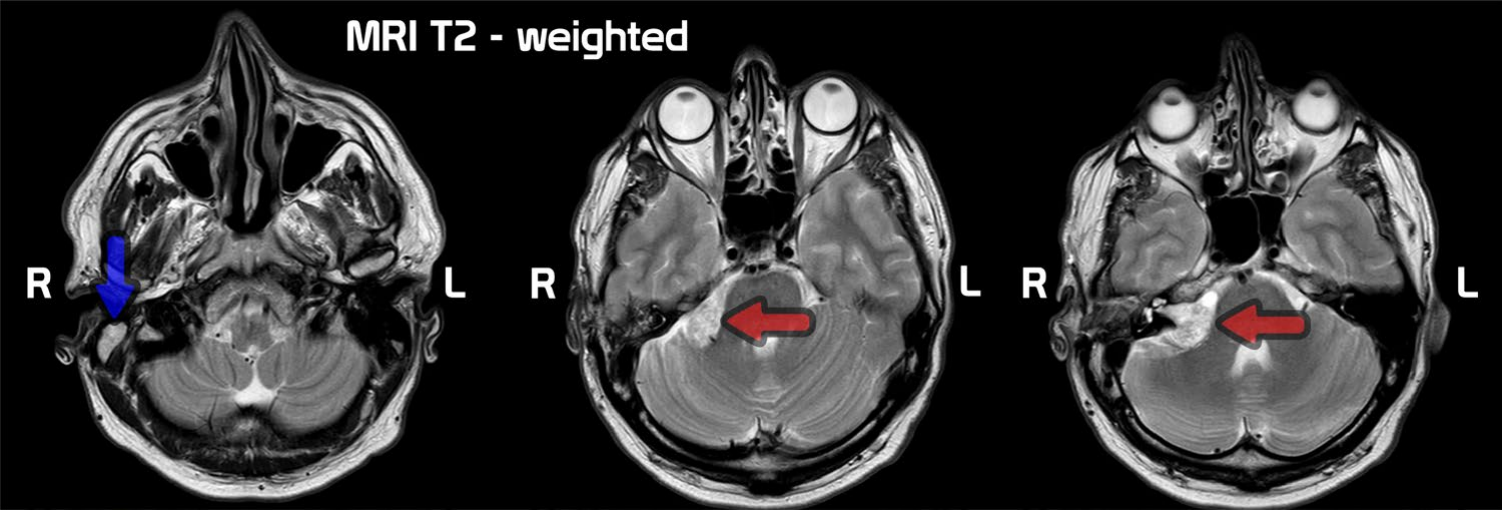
T2 MRI of the cerebellopontine angle (before surgery). Cholesteatoma of the tympanic cavity and mastoid (blue arrow). Right CP-angle tumor (red arrow)
